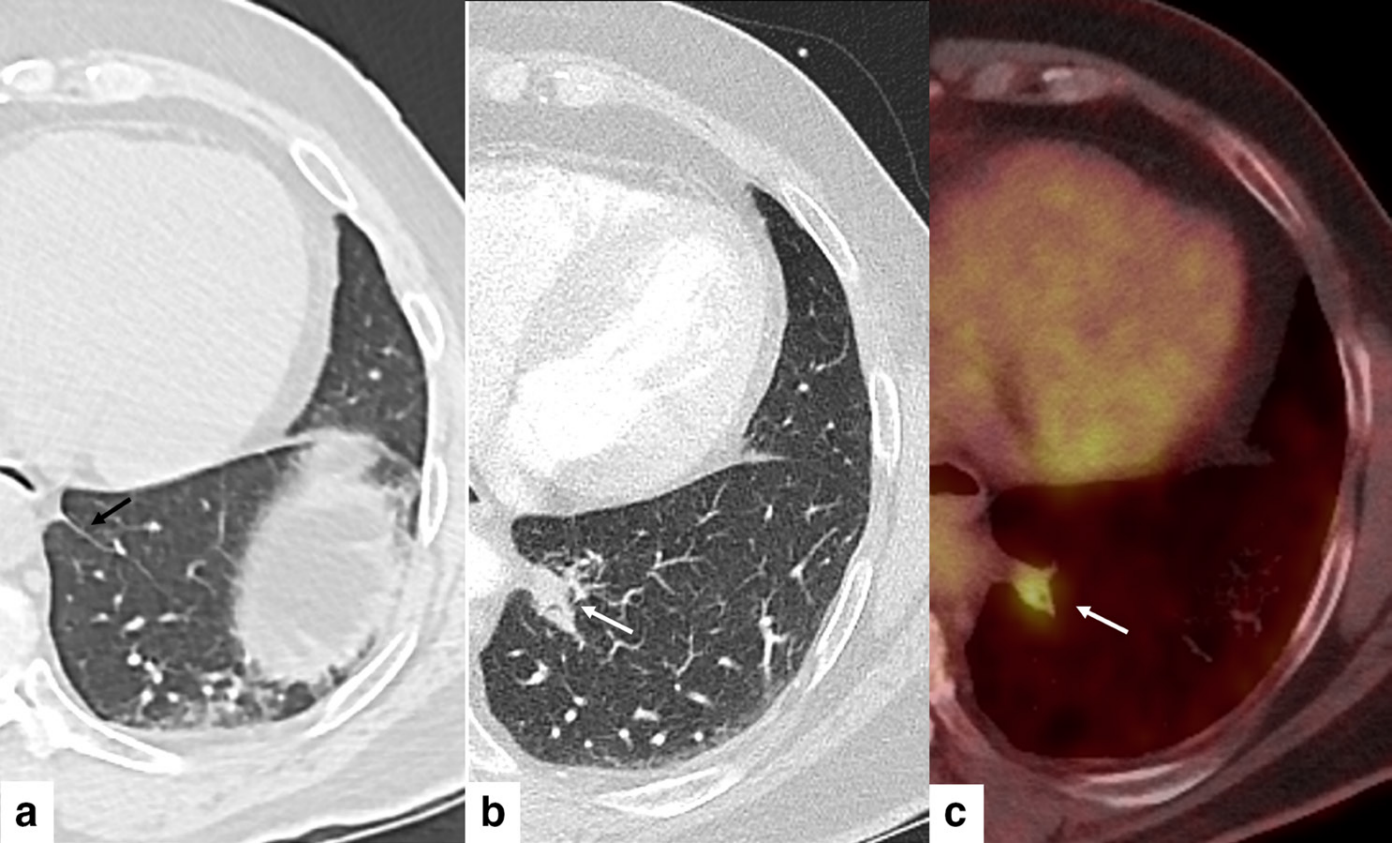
A-C. 65-year-old asymptomatic female with biopsy-proven granulomatous lymphadenitis. 4A: Baseline chest CT on lung windows showing a normal left IPL (black arrow). 4B: Repeat chest CT on lung windows performed 7 months later as part of aortic aneurysm surveillance showing a new lobulated 2.6 cm nodule within the left IPL (white arrow). 4C: PET CT demonstrated FDG-avidity with SUV of 4.6 within the nodule (white arrow), interpreted as “suspicious for malignancy”.
